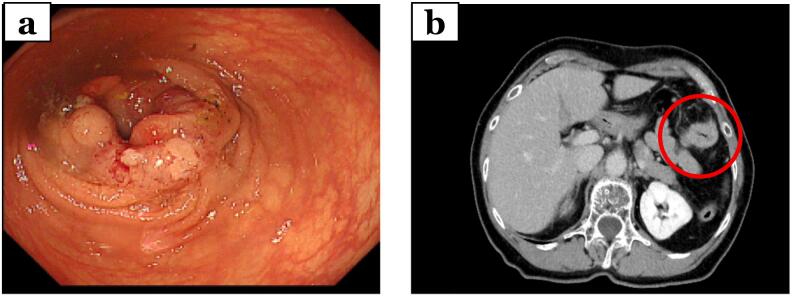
Image findings for diagnosis of transverse colon cancer. (a) Colonoscopy revealed a type 2 tumor in the transverse colon. (b) Computed tomography (CT) revealed a 5 cm mass in the splenic curvature of the transverse colon.
